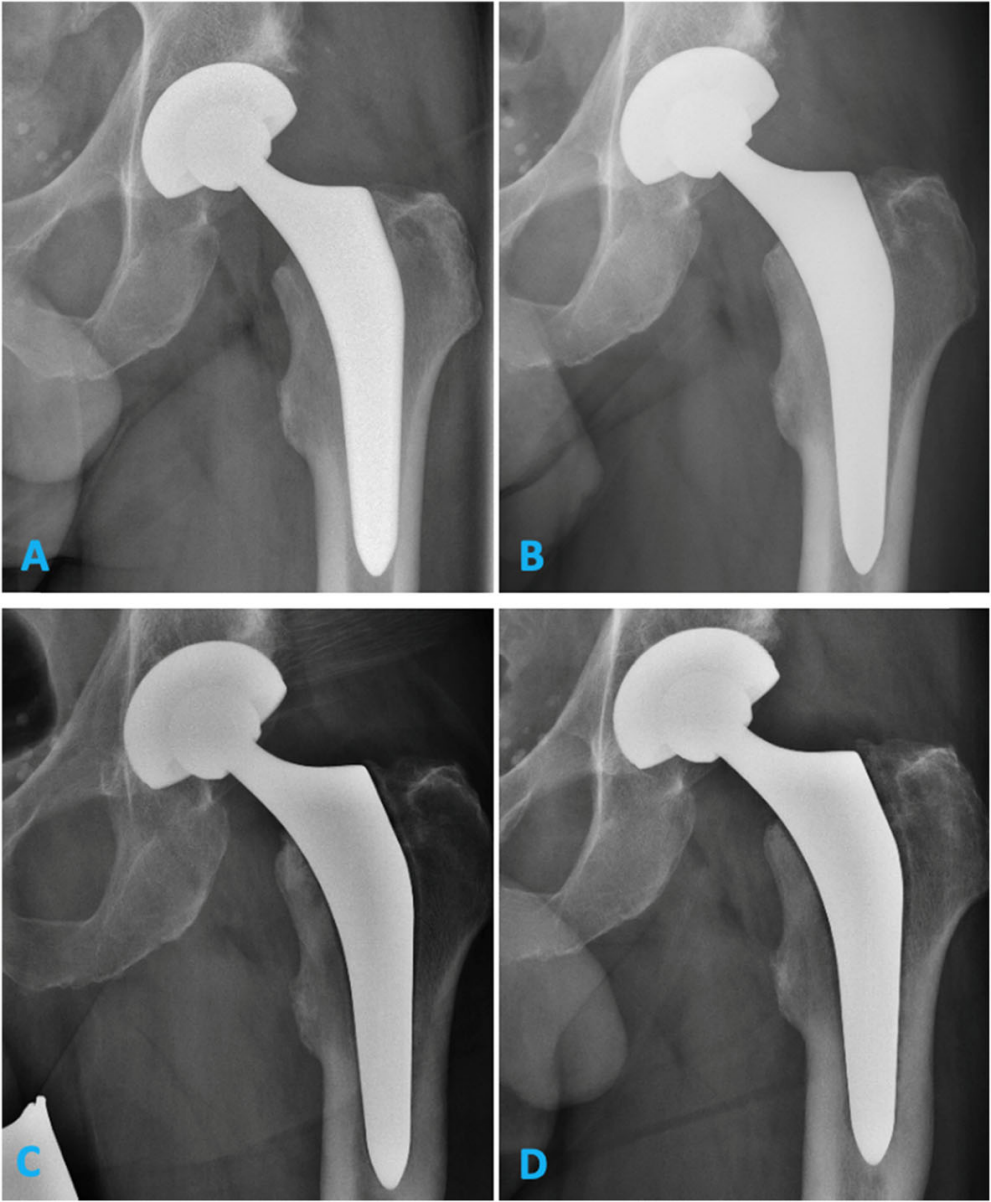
Anterior-posterior radiograph showing progressive periprosthetic radiolucency of the patient with aseptic loosening at 3 months (a), 1 year (b), 3 years (c), and 4 years (d) after surgery
